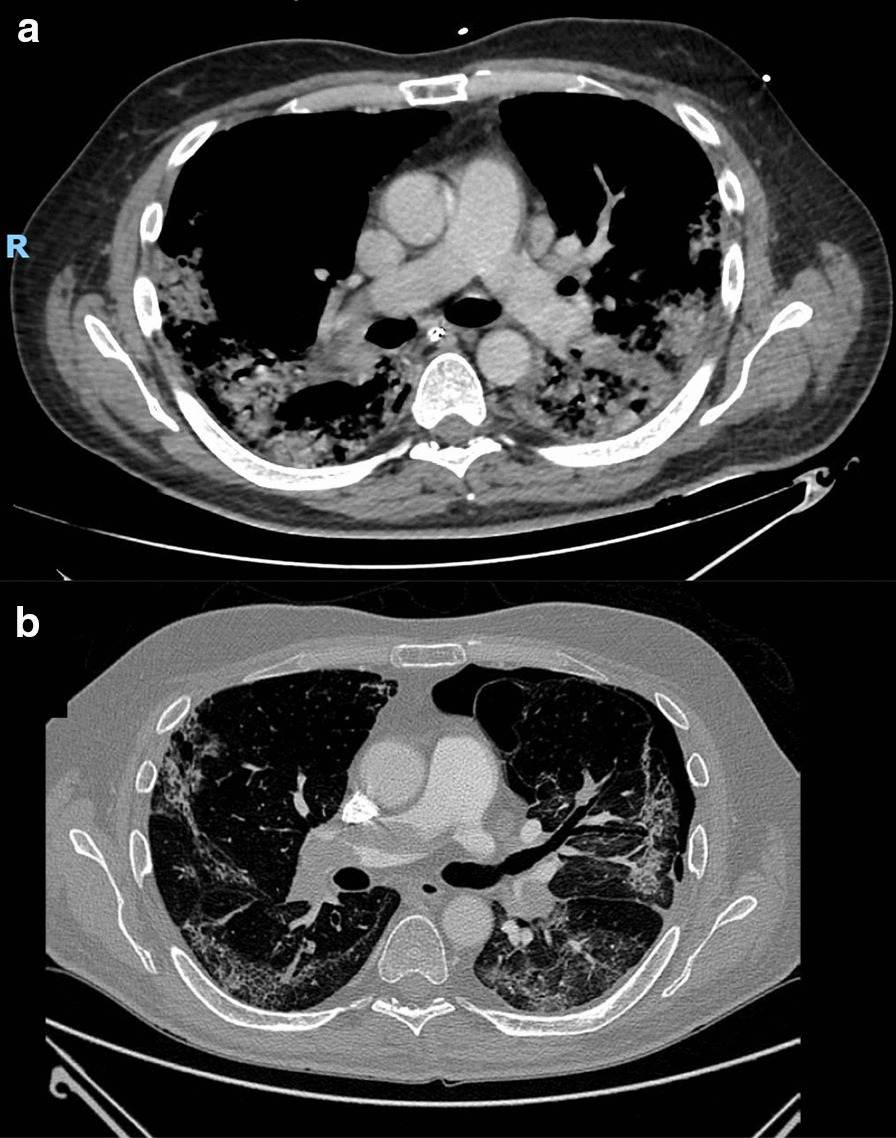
Computed tomography angiogram during the first hospital admission showing a focal filling defect affecting the pulmonary artery branch for the lower right lobe with thromboembolic outbreak (a). Computed tomography angiogram at the time of hospital re-admission showing extensive defect of endoluminal filling of the right and left pulmonary artery with extension to their main branches (b)
